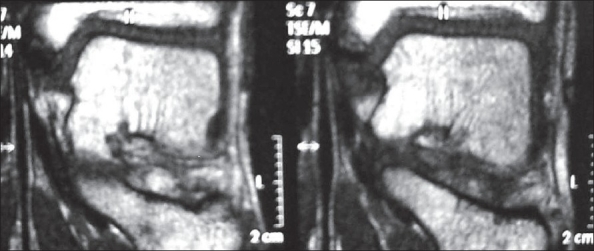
T2 WI MRI scan (coronal image) of the left side shows multifoci of hypointensity and fragmentation of the accessory center
